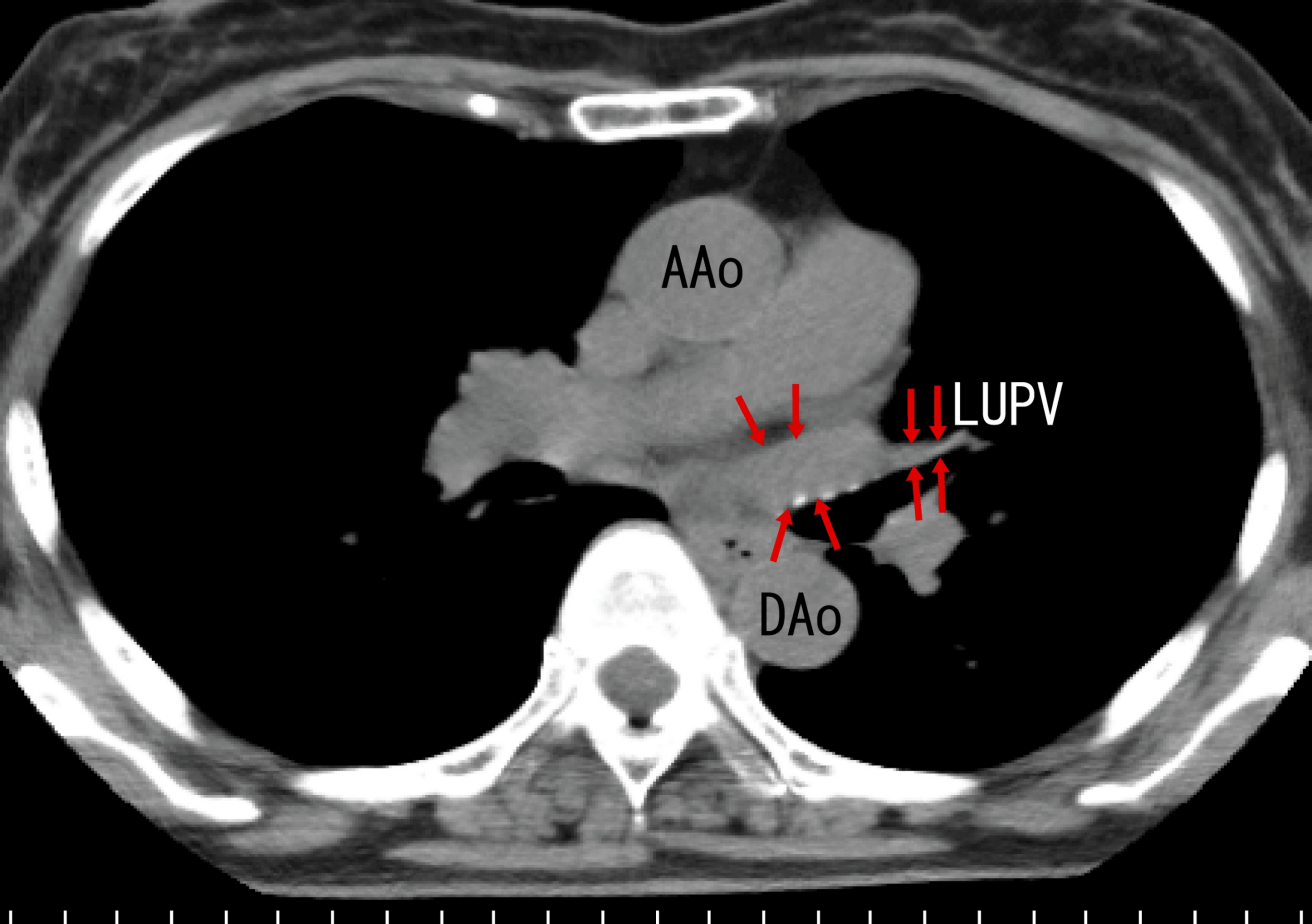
Axial plane CT images Axial images revealed no calcified thrombi in the LUPV as white areas (arrows); however, they revealed the cartilarge of the left bronchus as three white spots. AAo: ascending aorta, DAo: descending aorta, LUPV: left upper pulmonary vein
